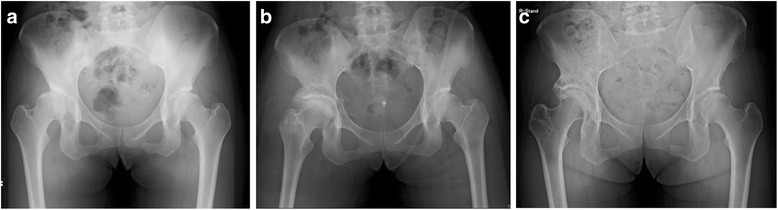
Radiographic image of a 24-year-old woman treated with rotational acetabular osteotomy on her right hip. Plane radiography of the hip showed an AHD, however, the joint space was persisting (a). The coverage of femoral head was improved after RAO (b). c is the radiograph at 12 years after RAO, she did not have any symptoms on her right hip and there was no progression of joint space narrowing
